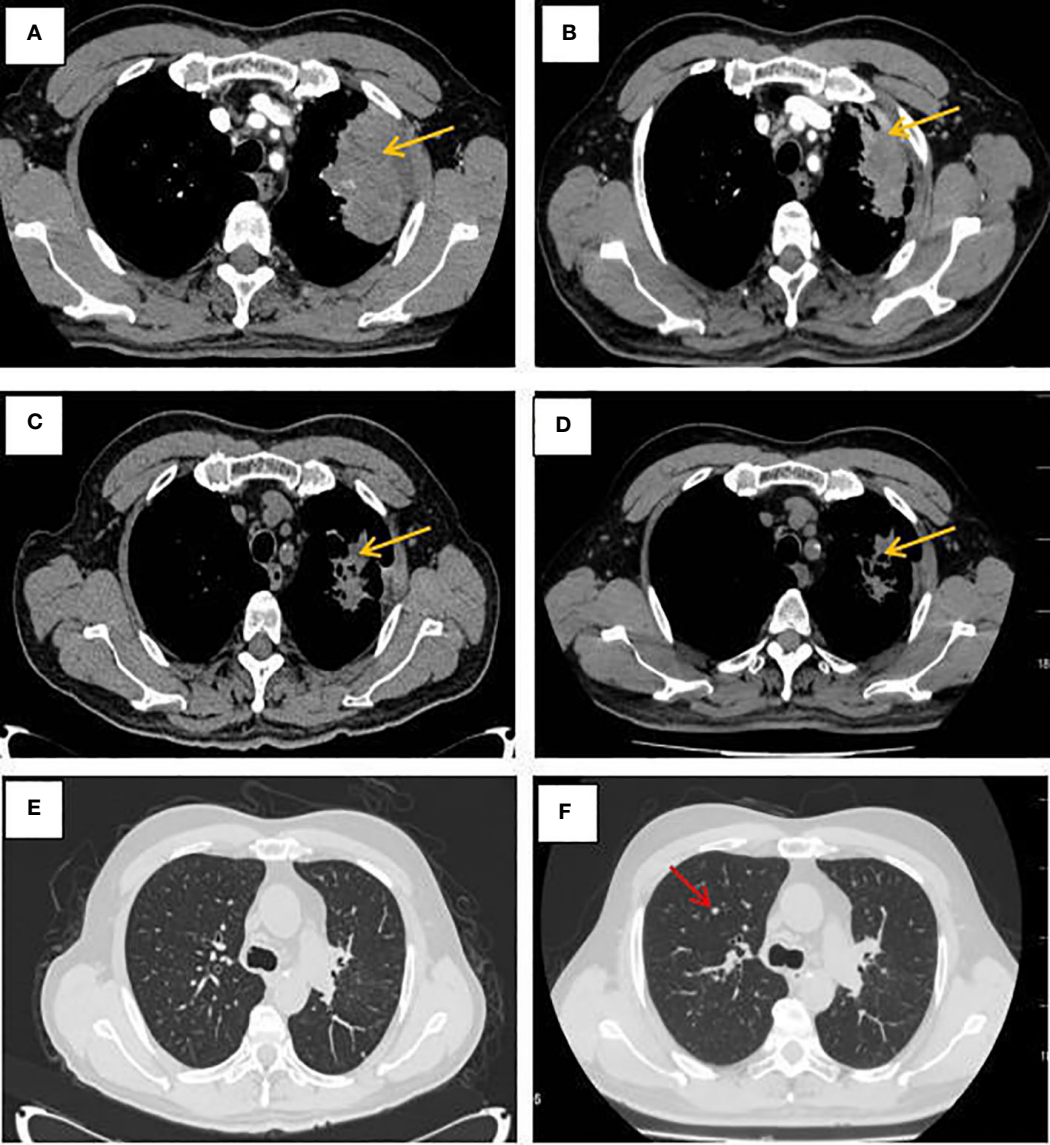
CT of the lungs at different times. (A–D) Mediastinal Window; (E–F) window of lung fields. (A) Pulmonary lesion before treatment. (B) CT revealed a stable disease after 2 cycles of albumin-bound paclitaxel and cisplatin in combination with sintilimab. (C) CT revealed a stable disease after 6 cycles of albumin-bound paclitaxel and cisplatin in combination with sintilimab. (D) CT showed no significant changes in the primary lesion of the left lung after sequential 2 cycles of sintilimab immune monotherapy maintenance treatment. (E)The window of lung fields after 6 cycles of albumin-bound paclitaxel and cisplatin in combination with sintilimab. (F) CT revealed pulmonary metastases increased after sequential 2 cycles of sintilimab immune monotherapy maintenance treatment (as the red arrow).
